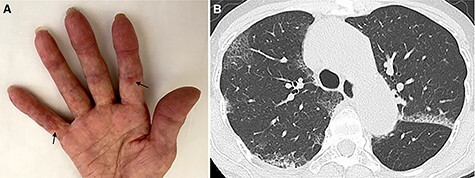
(A) The patient’s fingers with palmar papules (inverse Gottron’s sign; arrows). (B) Chest CT scan showed bilateral ground-glass opacities with a subpleural distribution.
